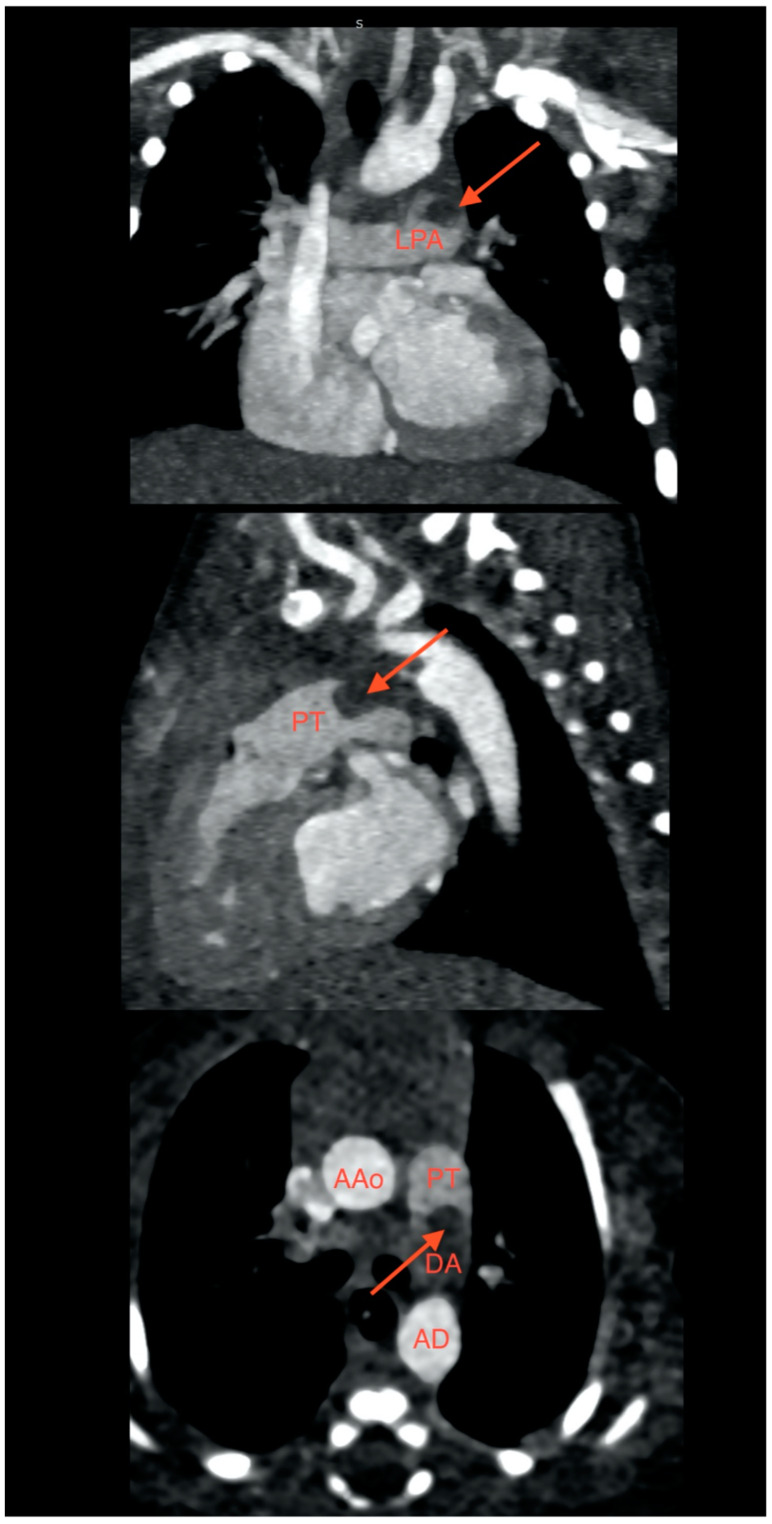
Angio-CT scan of 4-day-old neonate presenting thrombus extending into the left pulmonary artery, pulmonary trunk, and arterial duct. LPA: Left pulmonary artery; PT: Pulmonary trunk; DA: Arterial duct; AAo: Ascending aorta; AD: Descending aorta.
